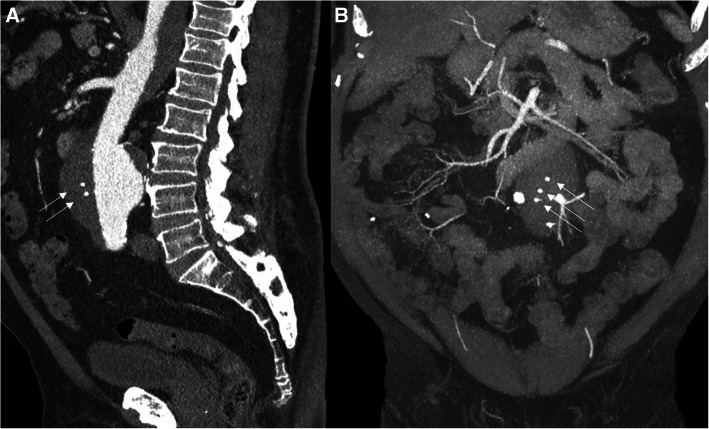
Maximum intension projection (MIP) reconstructions in the sagittal (a) and coronal (b) plane of the first post-procedural CT angiography showing markers of the plugs (arrows)
